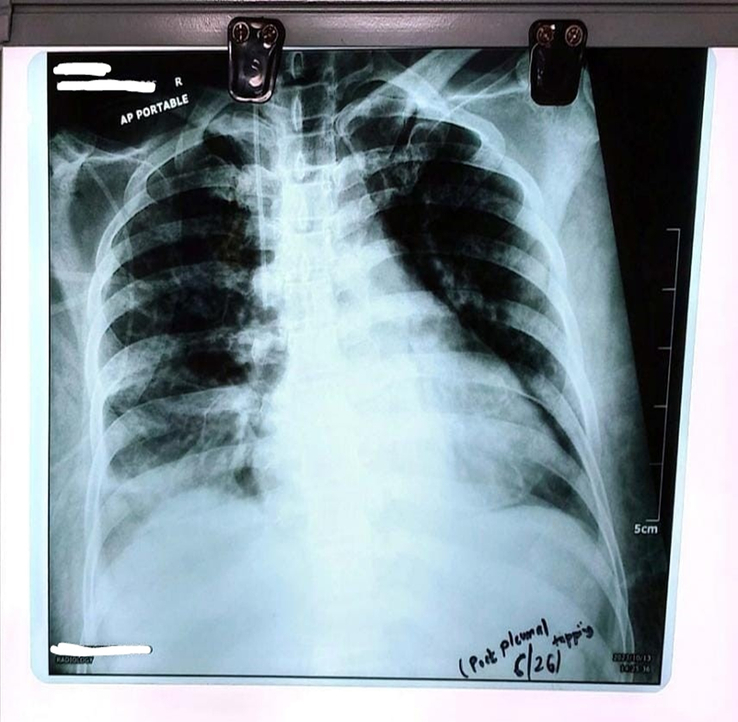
Chest radiograph anteroposterior view post-pleural tapping.
